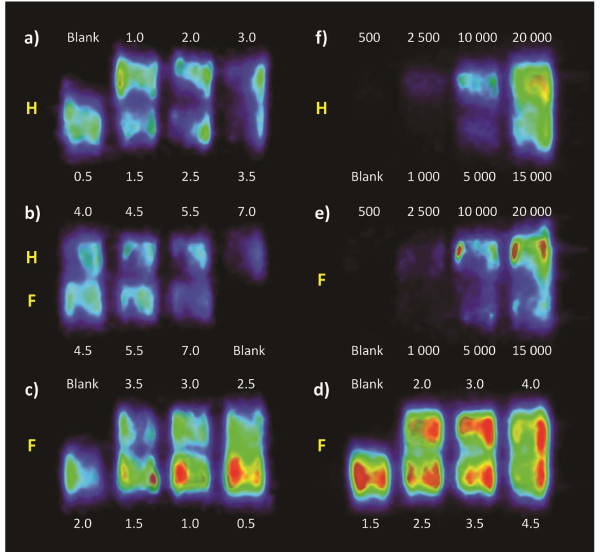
^18^FDG/PET images of HUVEC (H) and fibroblasts (F) pretreated with media containing glucose concentrations from 0.5 to 7.0 mM (a to c). Panel (d) shows a closer look at the plateau found with fibroblasts between glucose concentrations of 1.5 and 4.5 mM. Note that the color scale has been stretched in (d) to better appreciate the subtle differences between relatively similar samples. Numbers above and below the PET images represent the media glucose concentration in mM. Panels *e *and *f *show PET images of fibroblast and HUVEC monolayers at different cell densities treated with 3 mM glucose. Numbers above and below these images indicate the numbers of cells at seeding (8 hours prior to scanning).
